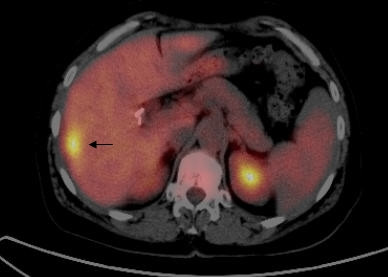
PET/CT fusion image on transaxial section demonstrates a hypermetabolic lesion (SUV_max _: 8.2, Patient number 14) in the segment 6 of the liver (arrow) which could not be detected by GP. Final pathology was consistent with CRC metastasis.
